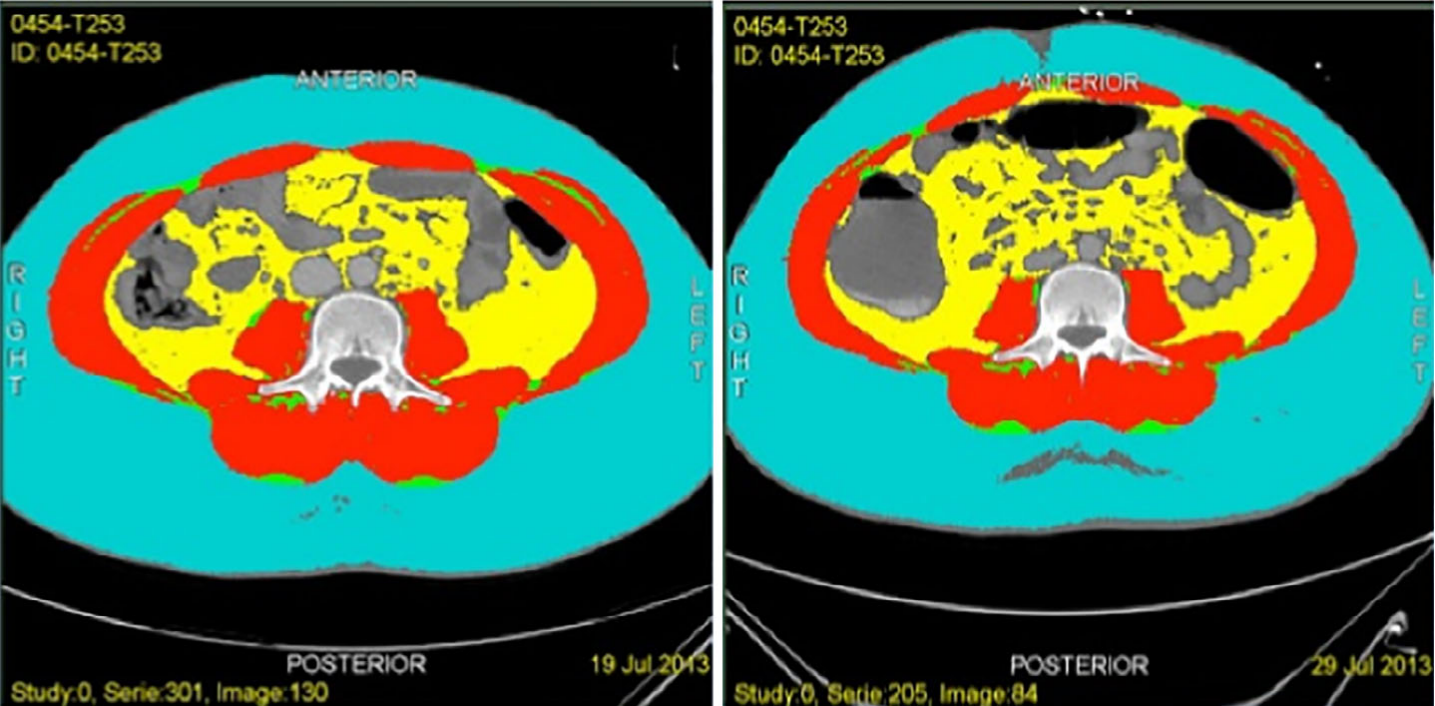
Visual loss of skeletal muscle on CT SMI
